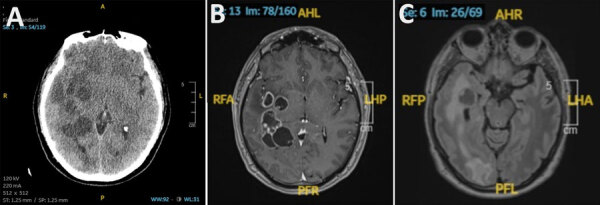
Radiologic findings from a study of autochthonous neurocysticercosis brain lesions mimicking metastatic disease, Spain. A) Noncontrast head computed tomography demonstrating multiple intra-axial lesions with surrounding vasogenic edema. B) Axial T1-weighted magnetic resonance imaging sequence with gadolinium showing multiple ring-enhancing lesions. C) Axial T2-FLAIR magnetic resonance imaging sequence revealing cystic lesions with internal nodular components suggestive of a scolex, surrounded by extensive edema.
